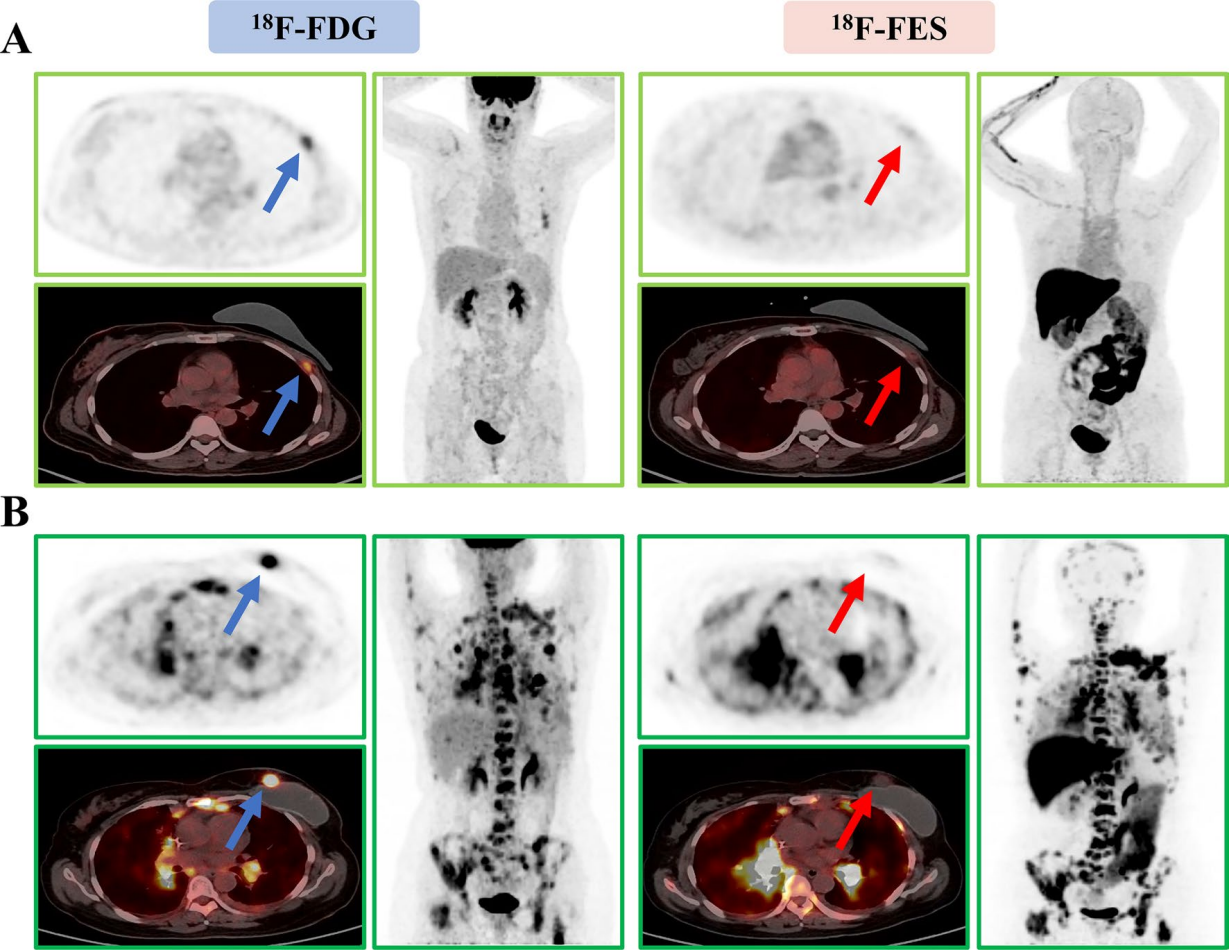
Representative imaging of patients with at least one ^18^F-FES-negative lesion. A Only ^18^F-FES-negative lesions (Fig. 2, #43). This 50-year-old woman had 4 ^18^F-FDG-positive lesions in her chest wall and lymph nodes, but all were negative on ^18^F-FES PET. She was on palbociclib combined with letrozole as first-line treatment for 2.3 months until progression occurred.  B Presence of ^18^F-FES-positive and ^18^F-FES-negative lesions (Fig. 2, #49). This 53-year-old woman had innumerable ^18^F-FDG-positive and ^18^F-FES-positive lesions in the pleura, lung, lymph nodes, and bone, but the left chest wall metastasis showed outstanding uptake of ^18^F-FDG but not of ^18^F-FES. She was on palbociclib combined with fulvestrant as third-line treatment for 5.6 months until progression occurred
